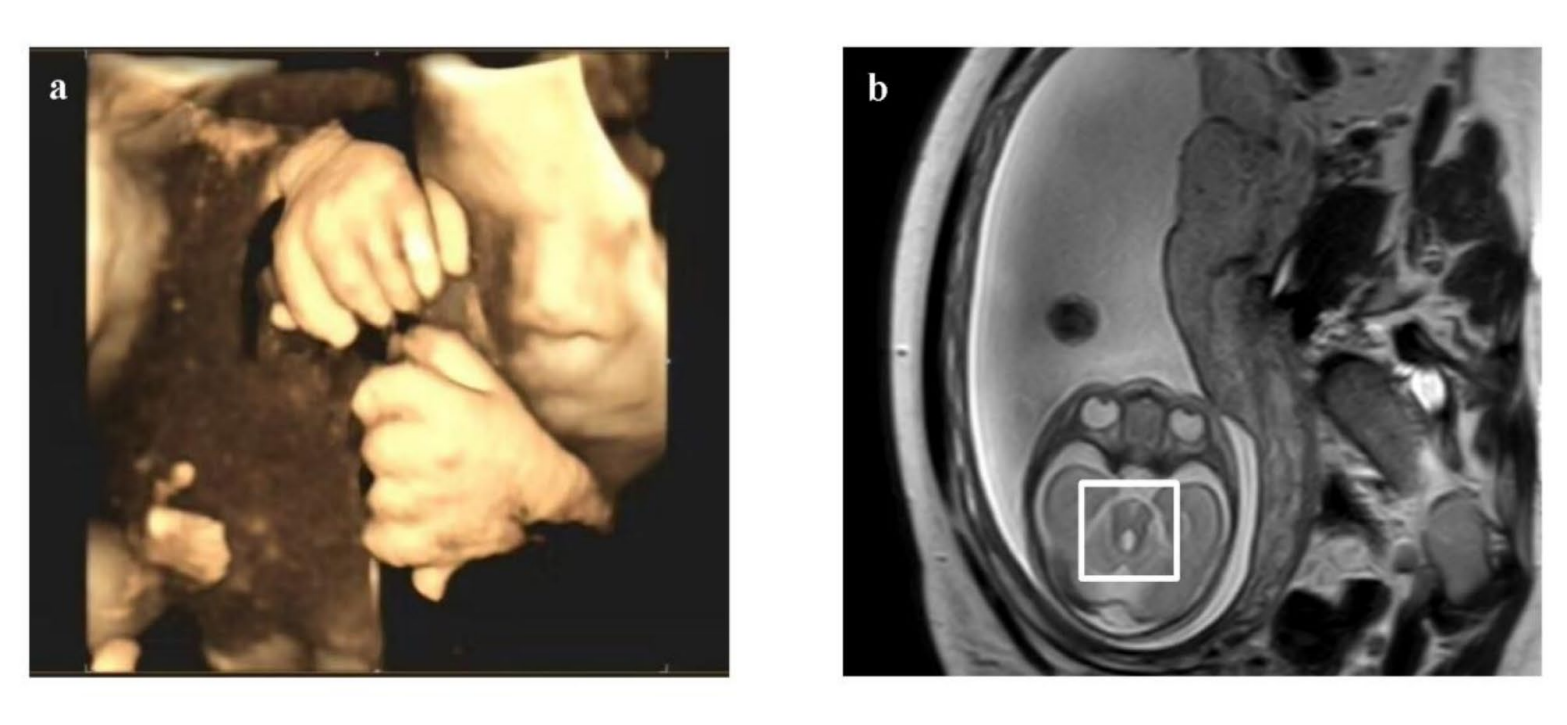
Imaging manifestations in *CPLANE1* variants as potential Joubert syndrome. (a) Polydactyly of both hands of the fetus on ultrasound at 33 + 1 weeks of gestation before abortion. (b) Partial agenesis of the vermis of the cerebellum and molar tooth signs on magnetic resonance imaging of the fetus at 25 + 1 weeks of gestation
